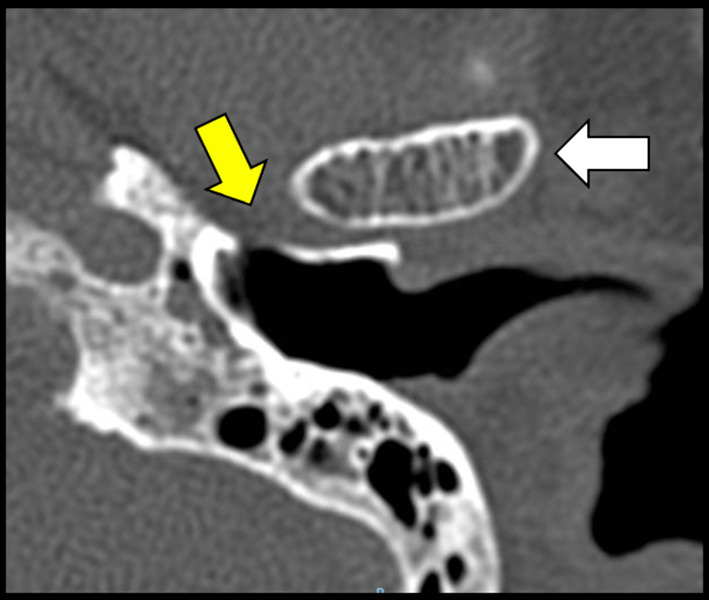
CT Axial view. Tympanic defect (yellow arrow) Mandibular condyle (white arrow)
